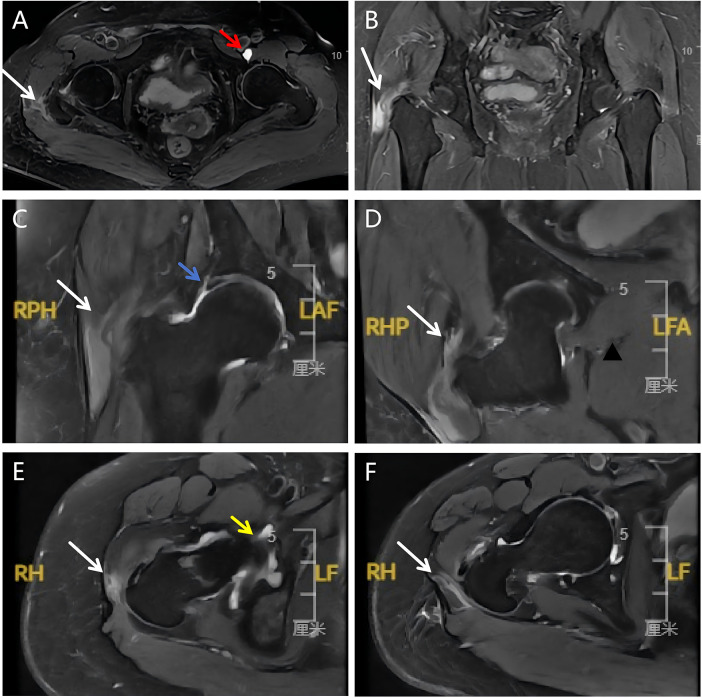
Representative MRI findings of hip rotator cuff injuries across different sequences and imaging planes. (A) Axial fat-suppressed MRI of both hips shows hyperintense signal around the right greater trochanter (white arrow), indicating a hip rotator cuff tear. A hyperintense labral cyst is present in the left hip (red arrow). (B) Coronal fat-suppressed MRI of both hips demonstrates marked hyperintensity around the greater trochanter (white arrow) with discontinuity of the gluteus medius tendon at its insertion, indicating a full-thickness tear. (C,D) Coronal and oblique coronal fat-suppressed MRI of the affected hip show heterogeneous signal at the gluteus medius insertion on the greater trochanter apex (white arrows), indicating a hip rotator cuff injuries. A linear hyperintense cleft between the acetabular labrum and acetabulum (blue arrow) suggests a labral tear. (E) Oblique sagittal fat-suppressed MRI of the hip demonstrates edema-like hyperintensity at the gluteus medius tendon insertion of the hip rotator cuff (white arrow), suggesting hip rotator cuff edema. Associated joint effusion is also present (yellow arrow). (F) Axial proton density–weighted fat-suppressed MRI shows hyperintensity at the gluteus minimus tendon insertion (white arrow), consistent with a gluteus minimus tendon tear.
